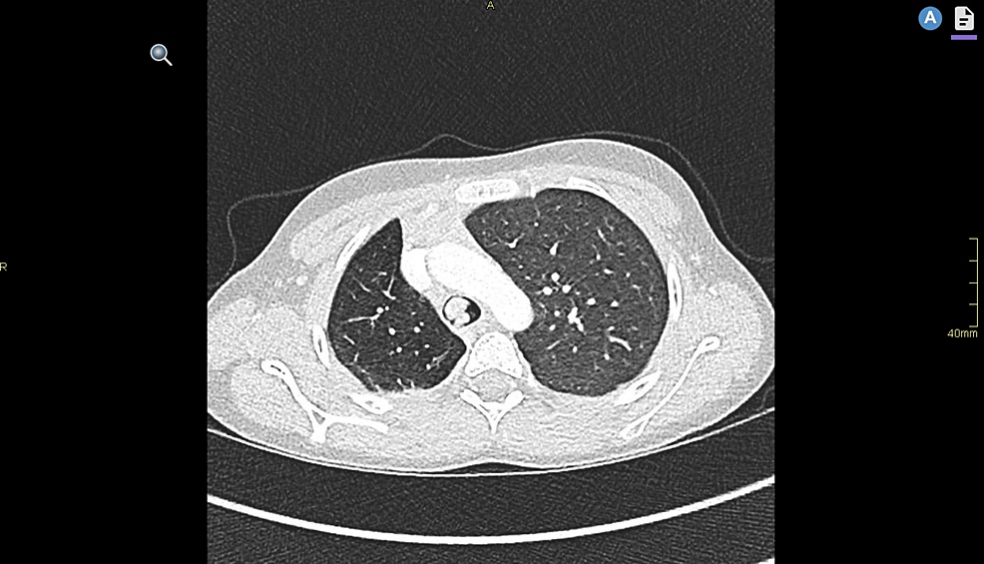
CT thorax axial section with contrast demonstrating a lobulated mass occupying much of the tracheal cross-sectional area
